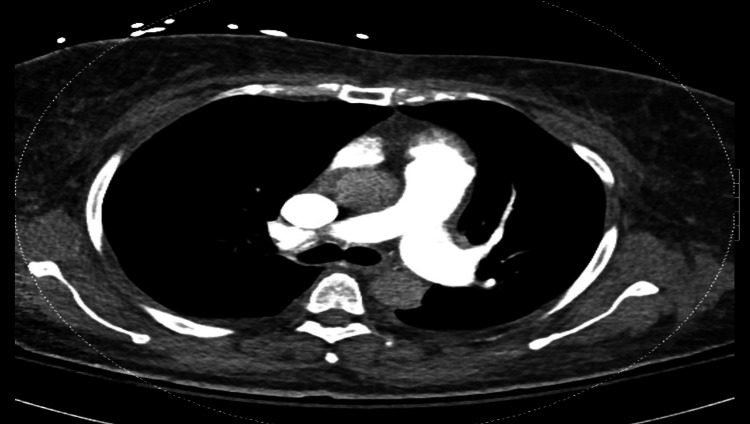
Preoperative CT showing enlarged PA CT: computed tomography, PA: pulmonary artery
